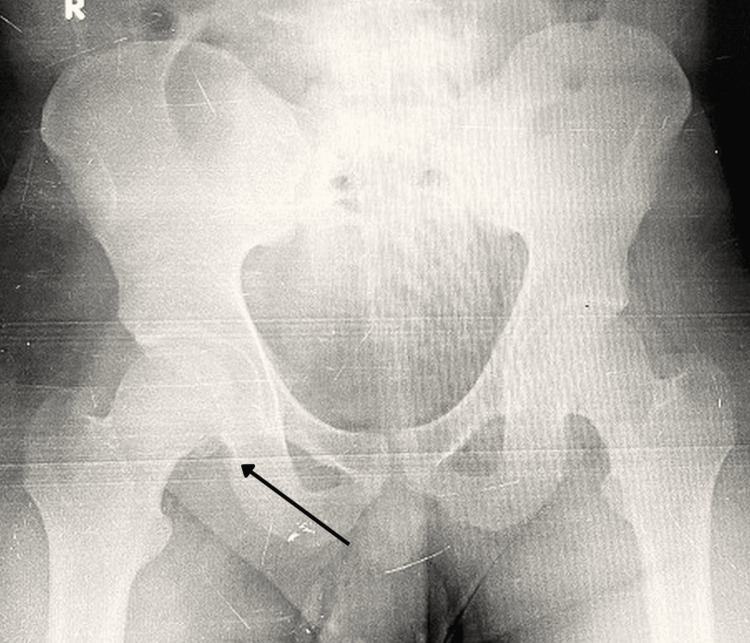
Post closed reduction anteroposterior radiograph of the pelvis with both hips. The arrow indicates the fracture site at the right femoral head, demonstrating concentric reduction of the femoral head within the acetabulum, with the Pipkin type II fragment located cranial to the fovea capitis. The femoral neck and acetabulum seem intact.
